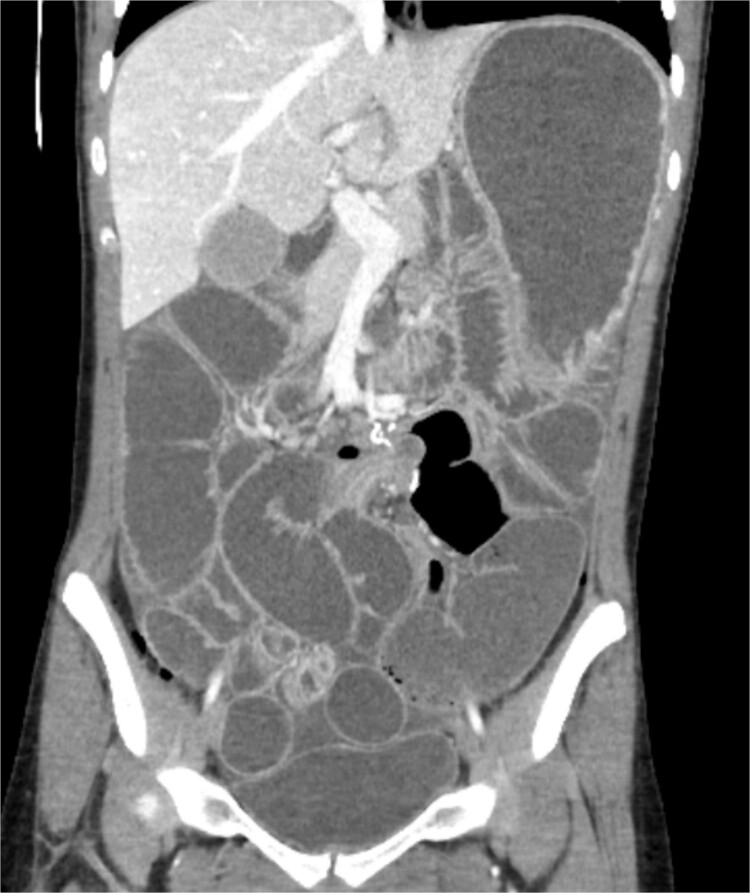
CT of the abdomen performed 8 days post operation. Shows proximal dilatation of the small bowel.
